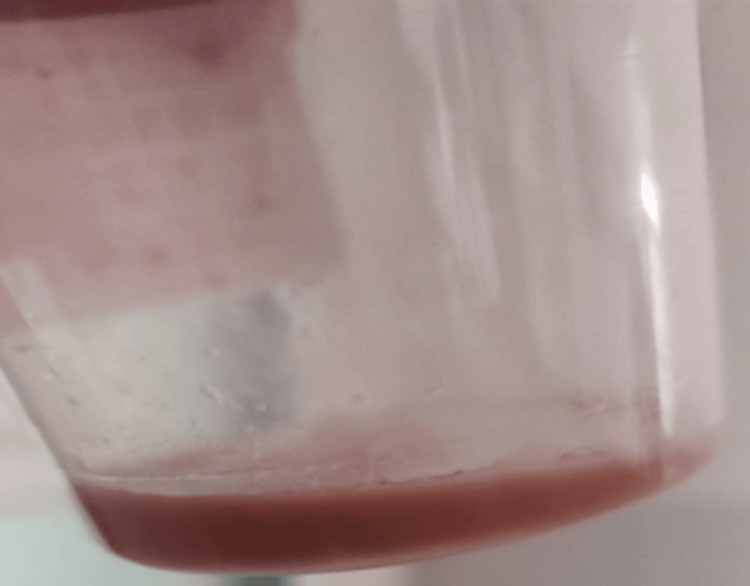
Macroscopic appearance of the psoas abscess aspirate. CT-guided needle aspiration yielded a thick, reddish-brown purulent fluid from the right psoas collection. The turbid and hemorrhagic appearance is highly suggestive of an active pyogenic infection.
